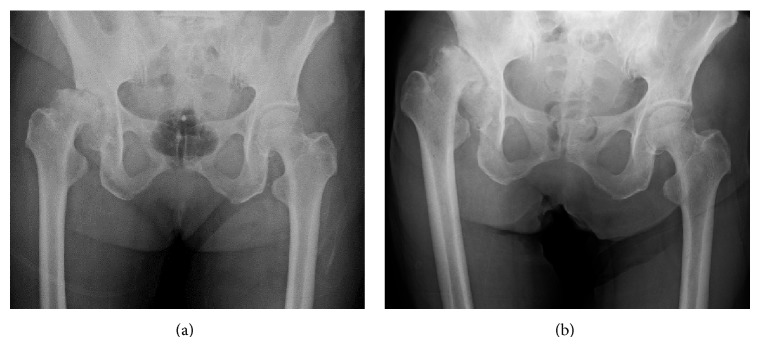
(a) A radiograph 2 months before admission to our hospital. (b) A radiograph 1 month after admission to our hospital. (a) and (b) show rapid hip joint destruction.
